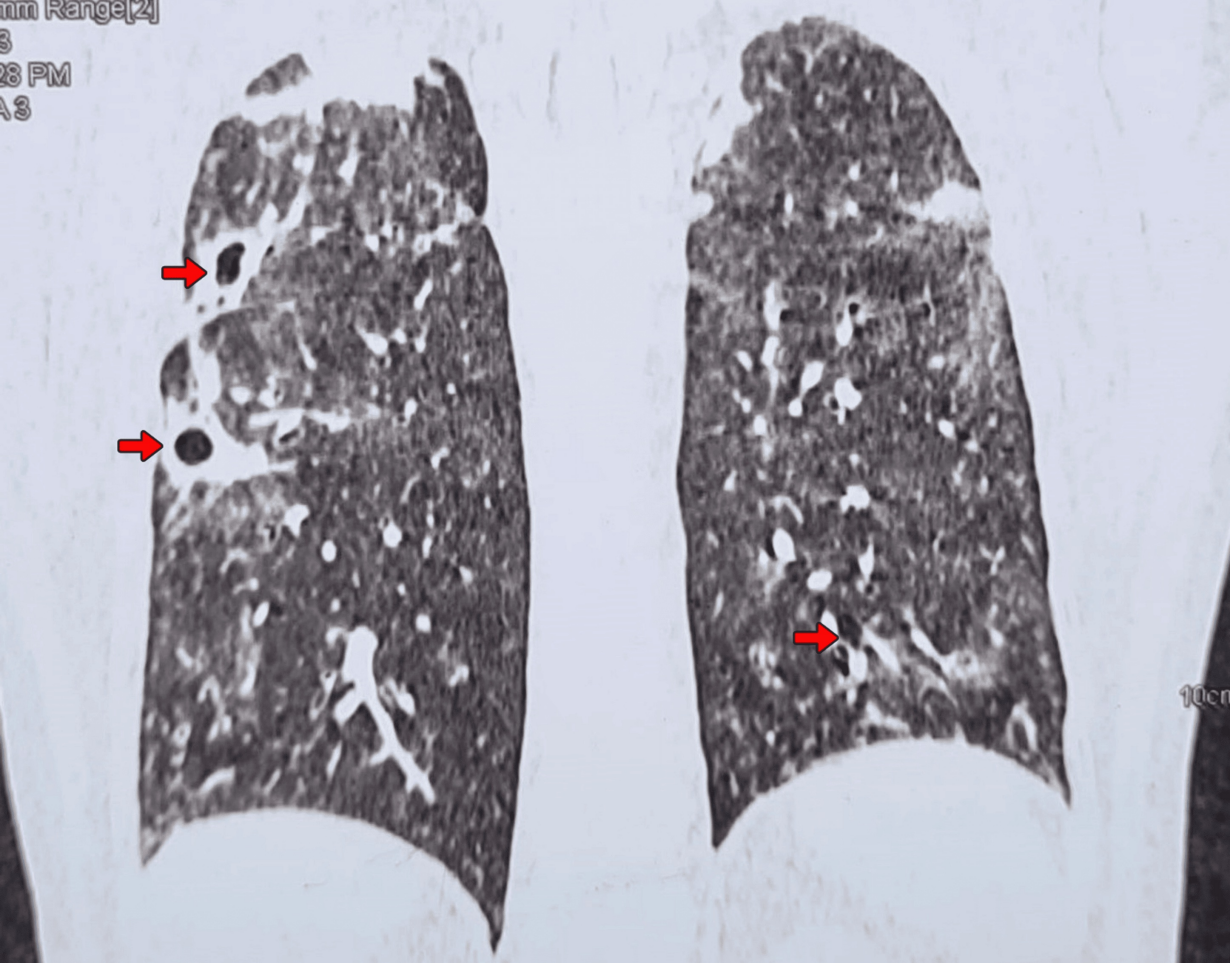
HRCT chest (coronal view) – cavitary lesions in upper lobes Coronal section of high-resolution computed tomography (HRCT) chest showing extensive bilateral upper lobe cavitations with surrounding ground-glass opacities (red arrows). These features support possible vasculitic or necrotizing pathology involving the pulmonary parenchyma.
